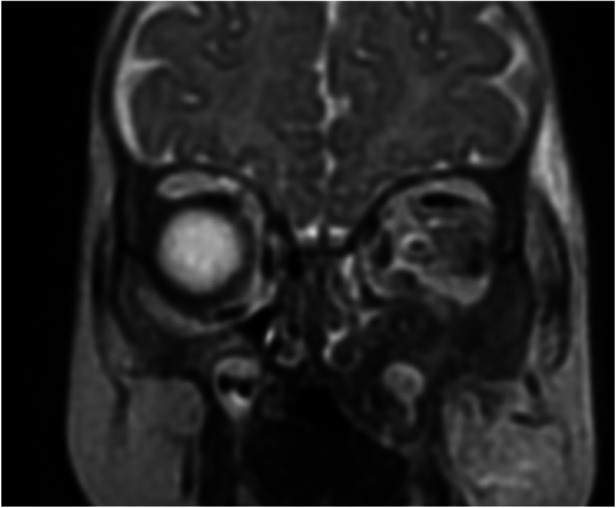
Coronal MRI of the paranasal sinuses shows an inflammatory process involving the left maxillary sinus, ethmoid sinus, floor of the orbit, and inferiorly into the sections of the hard palate.
